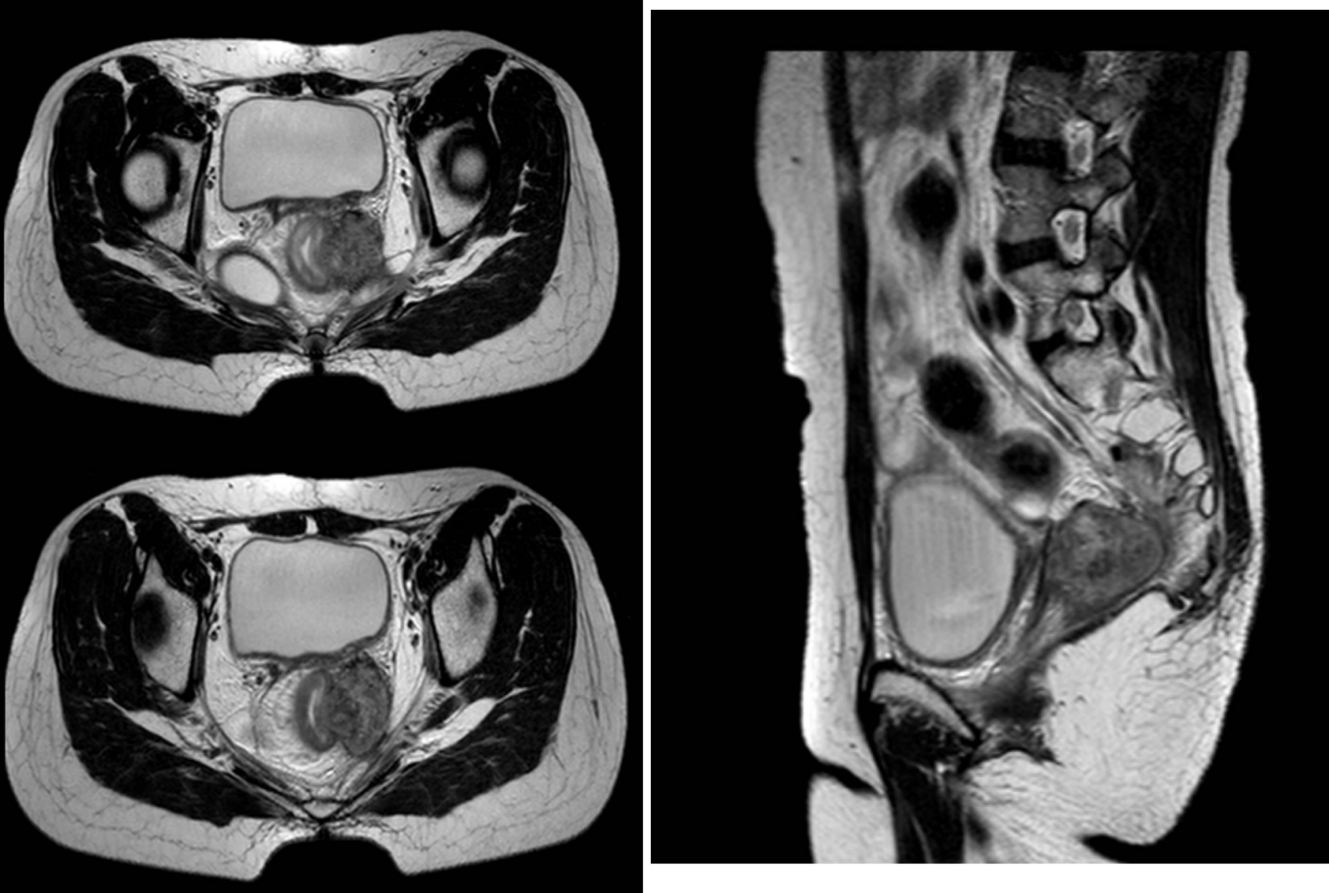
Pelvic MRI before reirradiation, recurrent tumors are shown in red circles.
